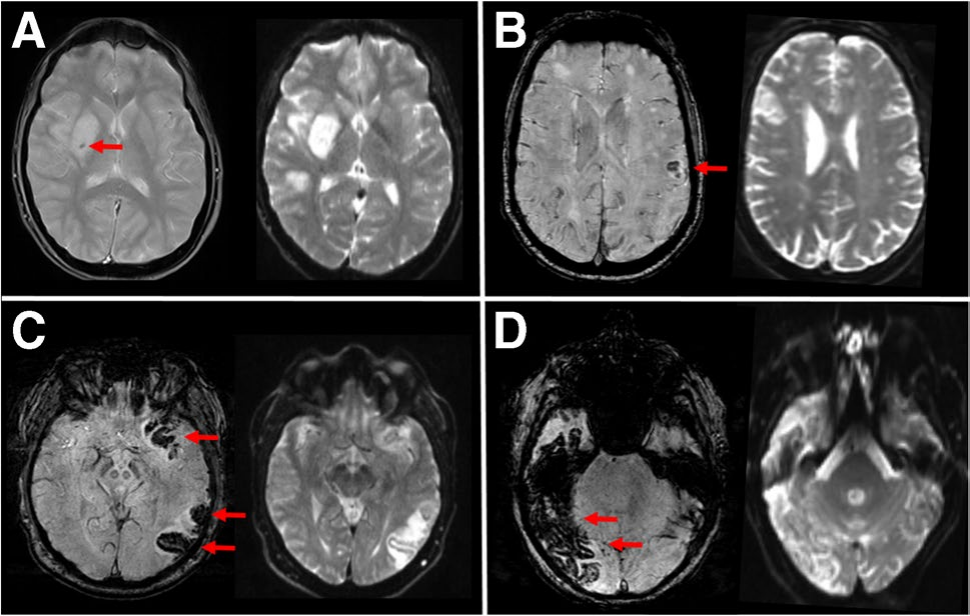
Different types of intracranial hemorrhages indicated by red arrows on T2*GRE/SWI but missed on DWI b0. (A) Small petechial hemorrhage classified as HI1 on T2*GRE at 1.5T but missed on DWI b0. (B) Small confluent hemorrhages classified as HI2 on SWI at 3T but missed on DWI b0. (C) Parenchymal hematoma classified as PH1 on SWI at 1.5T but missed on DWI b0. (D) Large parenchymal hematoma classified as PH2 on SWI at 1.5T but missed on DWI b0
